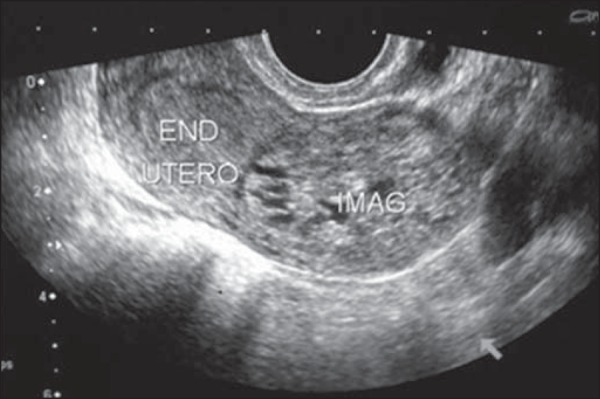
Transvaginal ultrasound showing an empty endometrial cavity, adjacent
